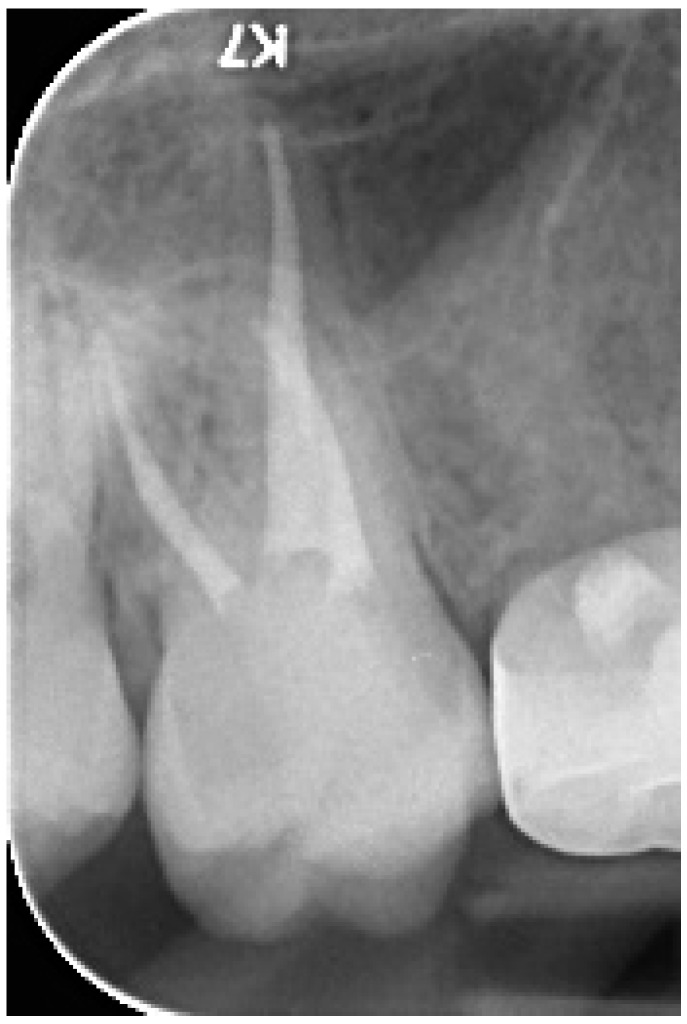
An X-ray after the completion of root canal treatment in the upper right first molar.
